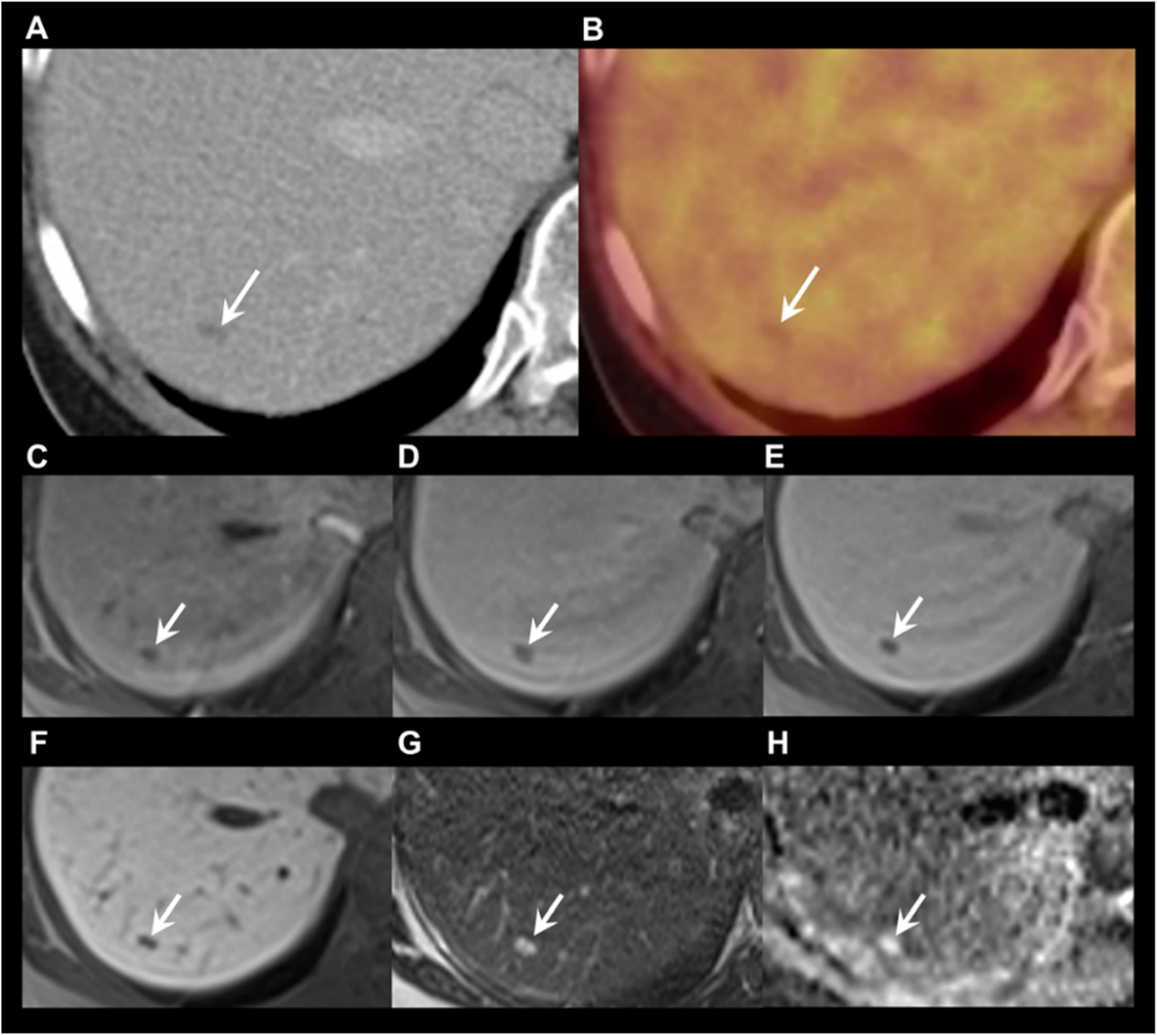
Lesion in segment VII with a change in categorization from category 2 on ^18^F-DOPA-PET/CT to category 1 through additional features on gadoxetic acid-enhanced MRI. a Contrast enhanced CT. b
